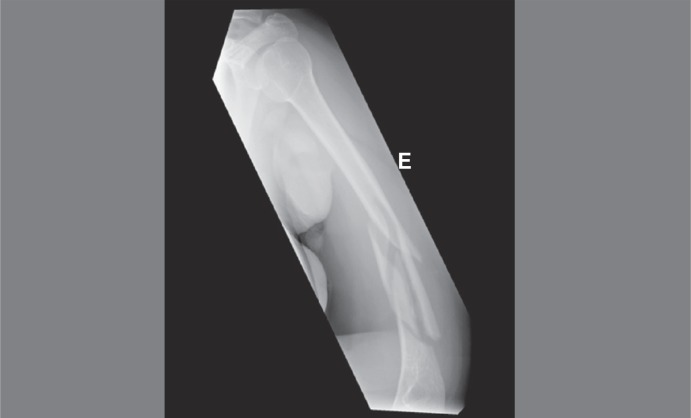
Preoperative X-Ray (Profile) of patient with fracture of the distal third of the humeral shaft
